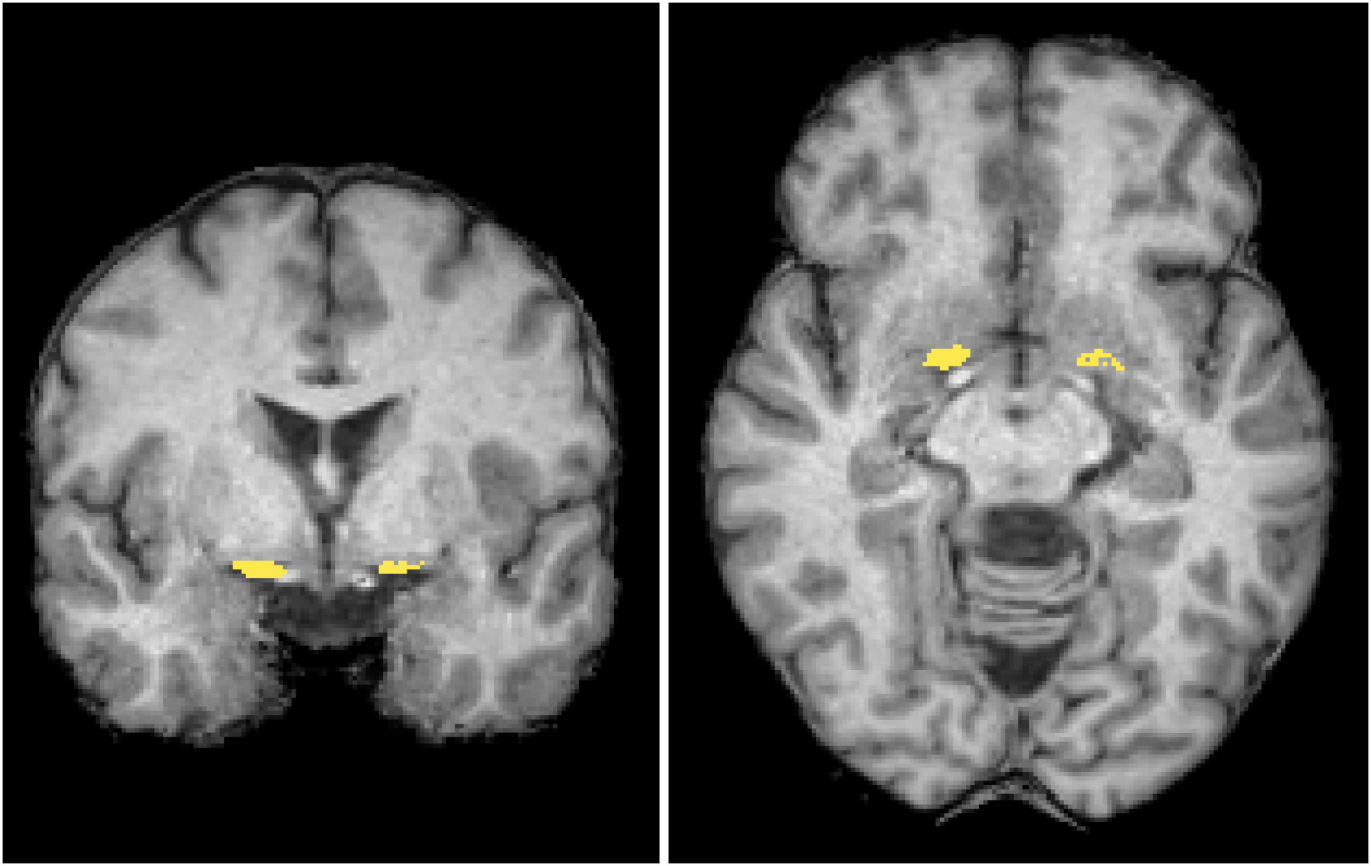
Regions of interest. The mask of the nucleus basalis of Meynert (NBM) (yellow) is overlaid in coronal and axial views on a standard brain T1-weighted images in the MNI space. MNI, Montreal Neurological Institute.
